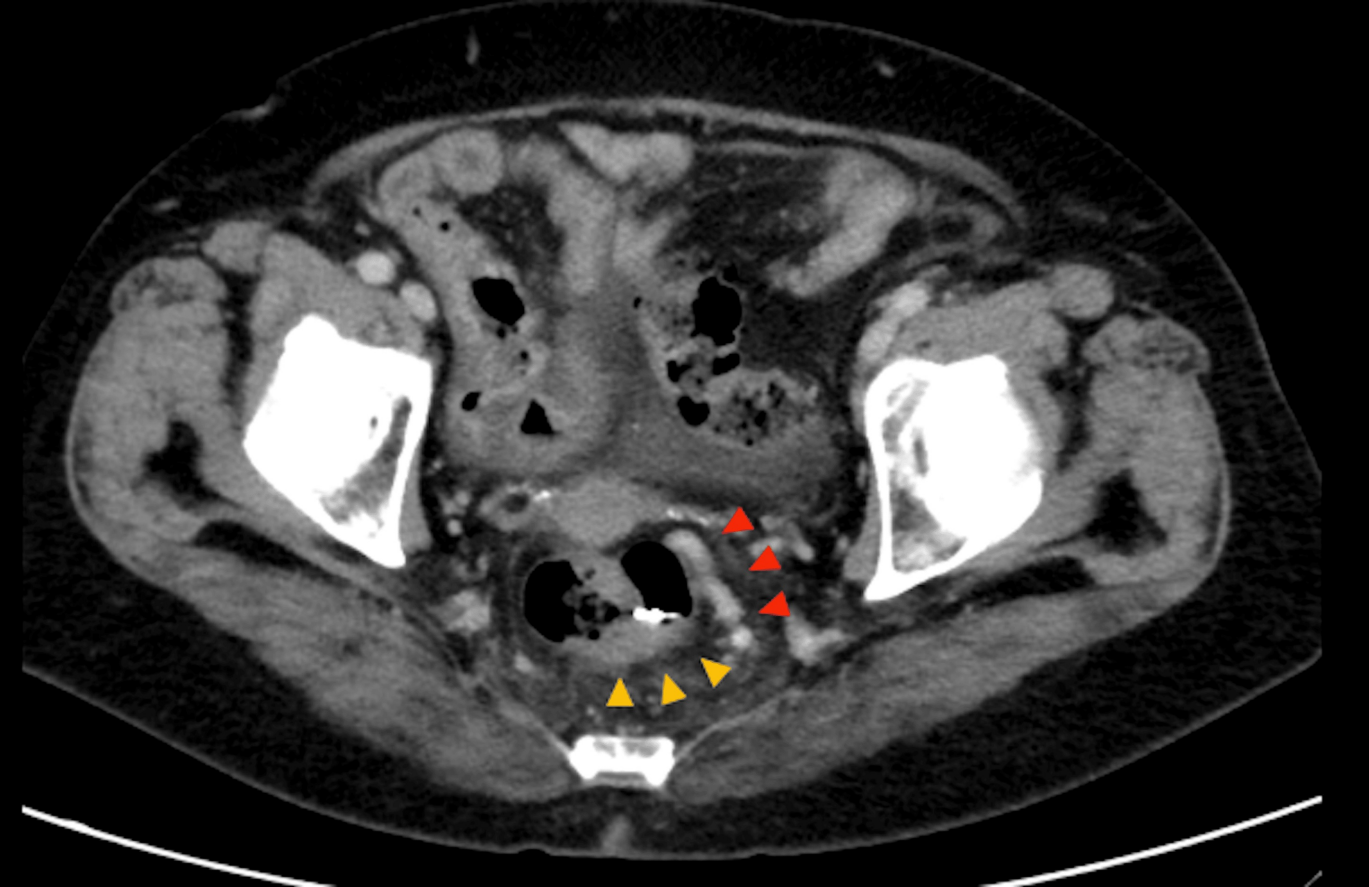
Computed tomography findings Image showing focal rectal wall thickening consistent with a primary tumor (yellow arrows), mild ascites, and prominent rectal varices (red arrows) protruding into the lumen due to portal hypertension without enlarged perirectal lymph nodes.
